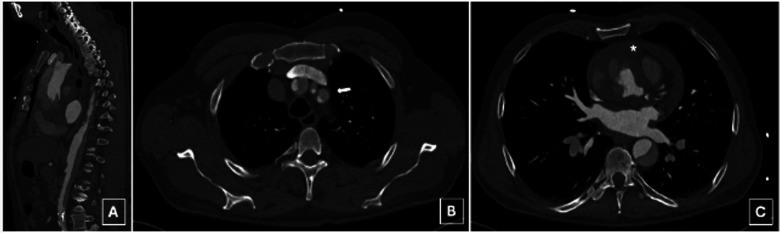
A preoperative computed tomography angiography disclosed an acute deBakey type I aortic dissection extending from the aortic root to the external iliac arteries (A), including supra-aortic branch (SAB) involvement (B, arrow) and hemorrhagic pericardial effusion (C, asterix).
